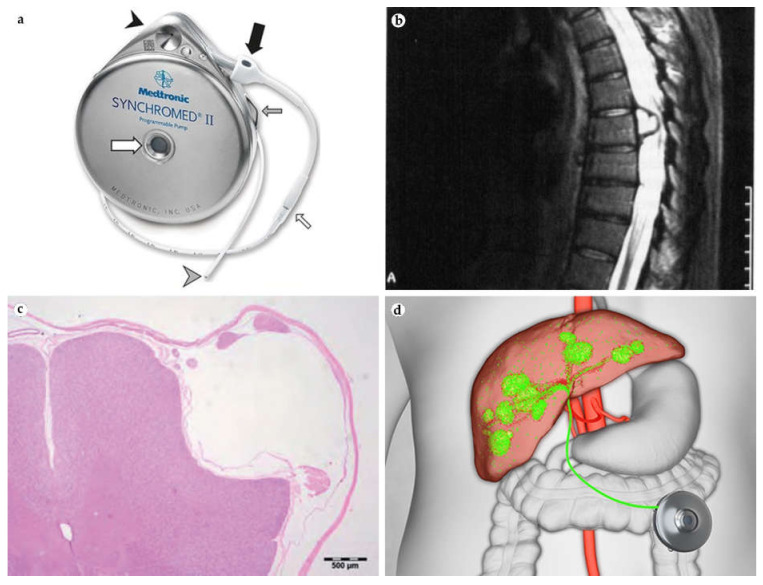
(a) External view of implanted Synchromed II pump and intrathecal catheter 8731SC. Pump with the catheter access port (black arrowhead), pump catheter connection (thick black arrow), refill membrane (thick white arrow) and suture loops for fixation (thin grey arrow), catheter–catheter segment connection (thin white arrow), and titanium catheter end (grey arrowhead). Reproduced from [69] under the terms of the Creative Commons CC BY license. (b) Gadolinium-enhanced T1 weighted sagittal MRI image showing extrinsic mass formed in the area of catheter insertion and compressing the spinal cord at T7 after implantation of Synchromed pump with permanent catheter (#8703) for the delivery of analgesic drugs. After 4.5 years of intrathecal drug delivery, the patient developed increasing neurological deficit and paresis. The pathology examination revealed a cyst containing sterile eosinophilic liquid material without leucocytes. The cyst capsule was infiltrated with lymphocytes. Reproduced from [34], with permission from WILEY. (c) Spinal cord compression caused by the catheter of a non-identified intrathecal pump IDDS without morphine elution. Note the evident compression of the spinal cord in the vicinity of the catheter track and absence of morphine-associated granuloma formation. Reproduced from [64], with permission from Oxford University Press and Copyright Clearance Center. (d) Scheme of hepatic artery infusion with Intera 3000. Reproduced with permission by Intera Oncology [70].
